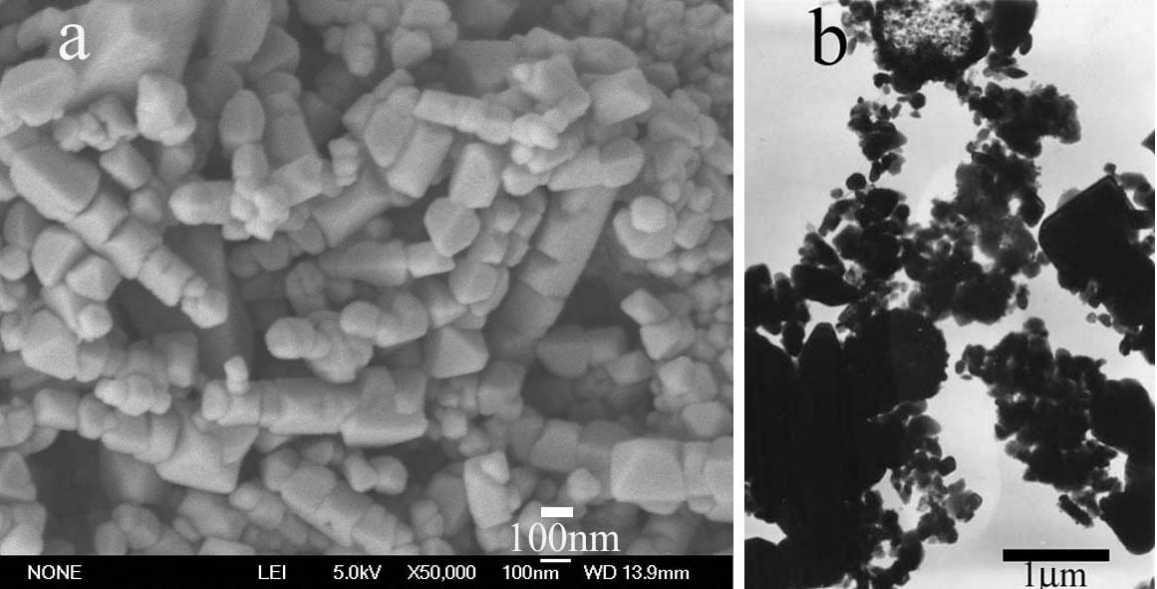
TEM or FESEM images of γ-Mn_3_O_4 _products obtained under different reaction conditions. a. FESEM image of 0.084 g MnSO_4_·H_2_O and 5.0 g CT at 210°C without thiourea. b. TEM image of 0.084 g MnSO_4_·H_2_O and 5.0 g CT at 210°C with 0.039 g urea.
